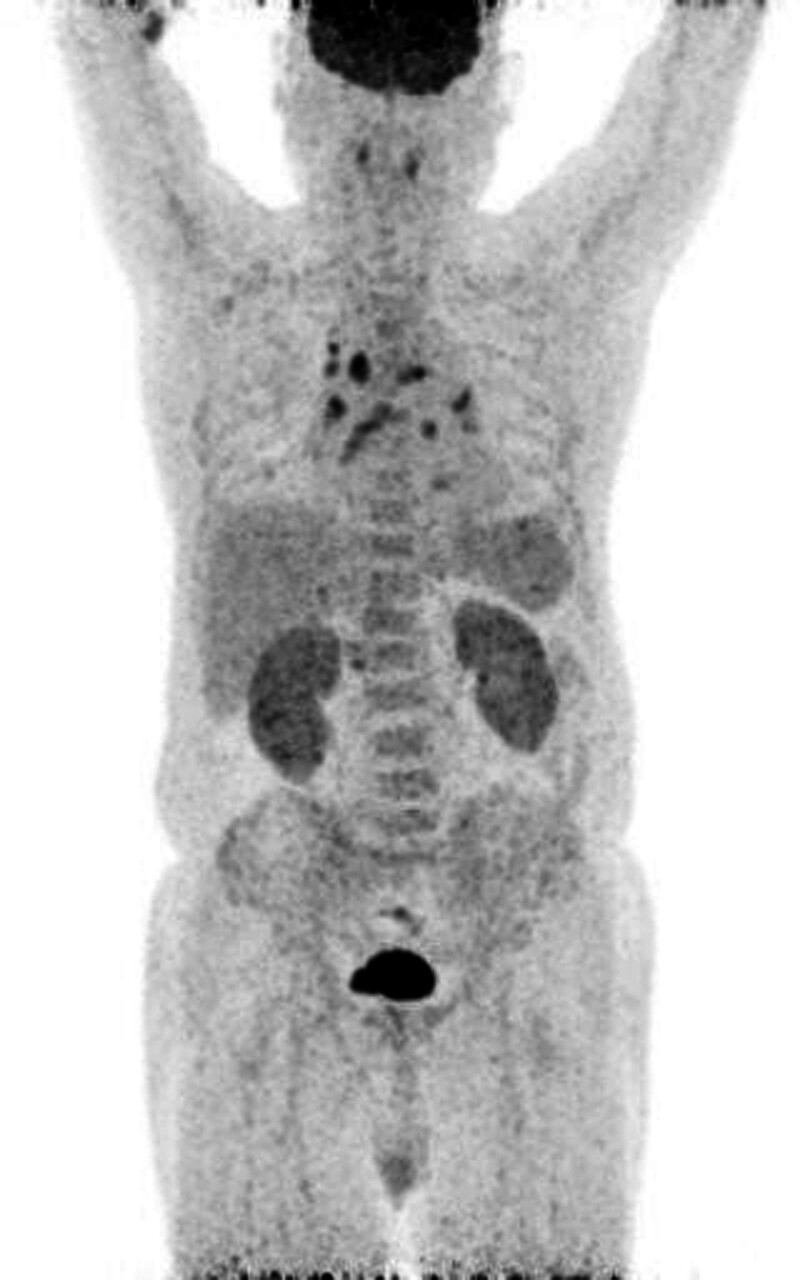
Fluorodeoxyglucose (FDG)-positron emission tomography scan of the torso. The presence of generalized heightened FDG uptake in both the bone marrow and spleen is noted, which prompted consideration of potential involvement of multiple myeloma. The mediastinal hypermetabolic lymph nodes 3A, 4R/L, 5, 6, 7, and 8R/L are considered reactive. FDG = fluorodeoxyglucose.
